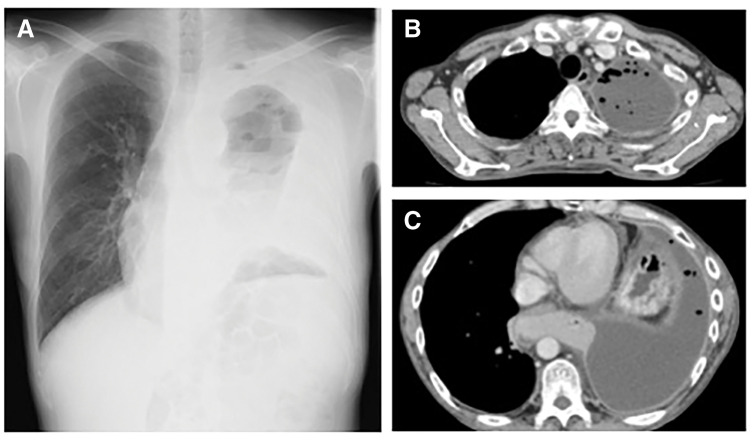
Imaging findings after naso-pancreatic drainage. (A) Chest radiograph showing persistent left pleural effusion with incomplete re-expansion of the left lung. (B, C) Follow-up contrast-enhanced chest CT demonstrating a loculated left pleural effusion with marked pleural thickening and incomplete re-expansion of the left lung, suggesting organized pleural inflammation and lung entrapment.
